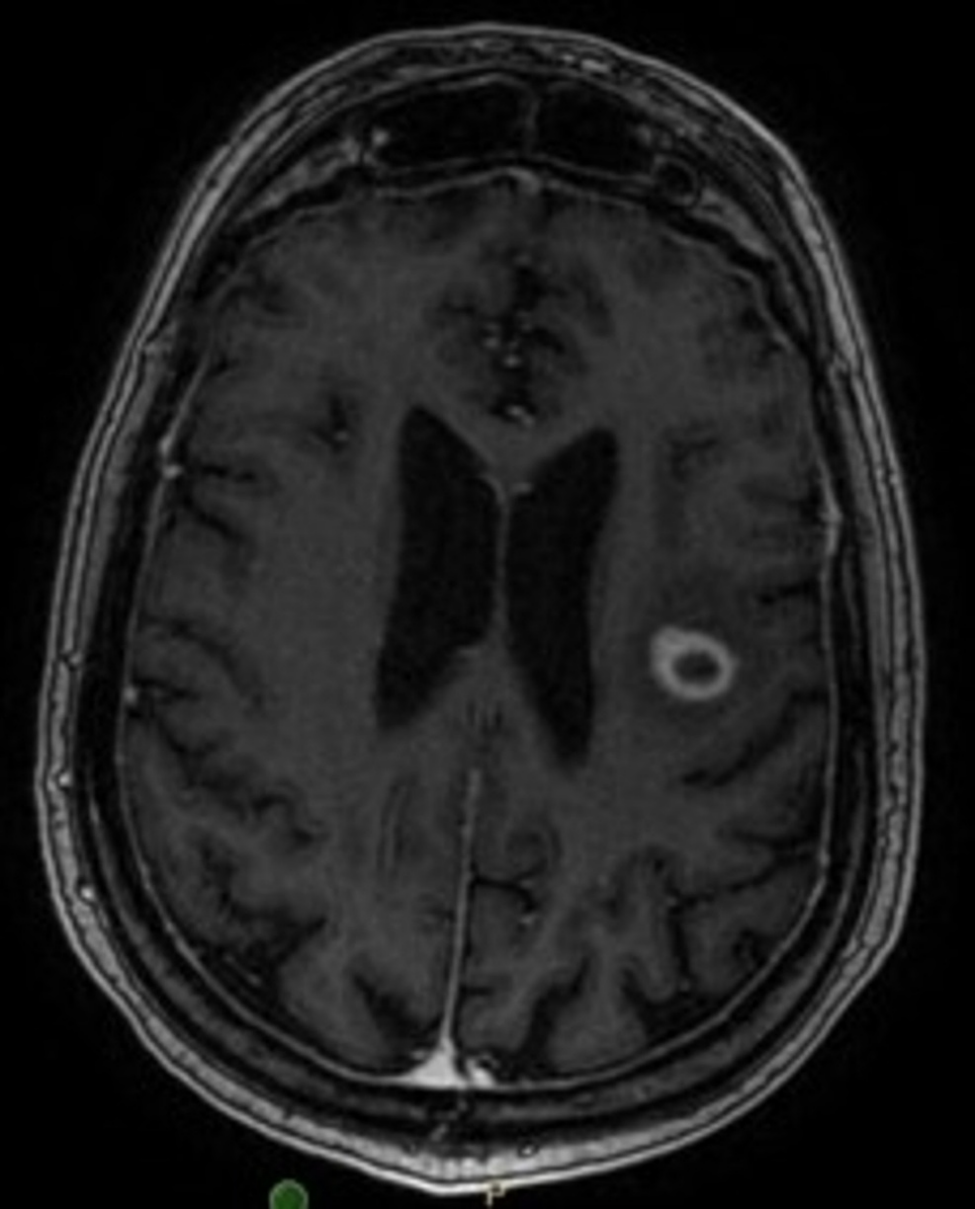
MRI brain with contrast, T1-weighted image showing 1.5 cm left frontal lobe lesion
